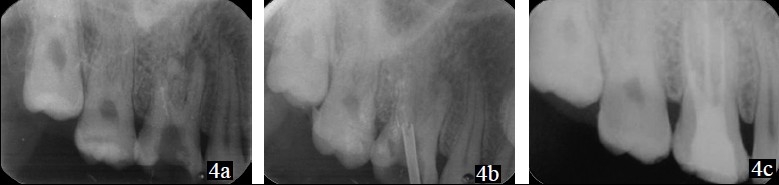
(a) – Radiograph showing the separated file in the distobuccal canal; (b) – Trephan centered over the fragment; (c) – Reobturated 16
